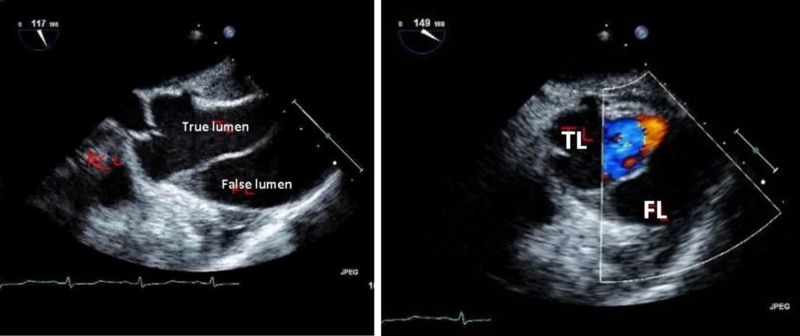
Transesophageal echocardiogram showing Stanford type A acute aortic dissection with true and false lumens
